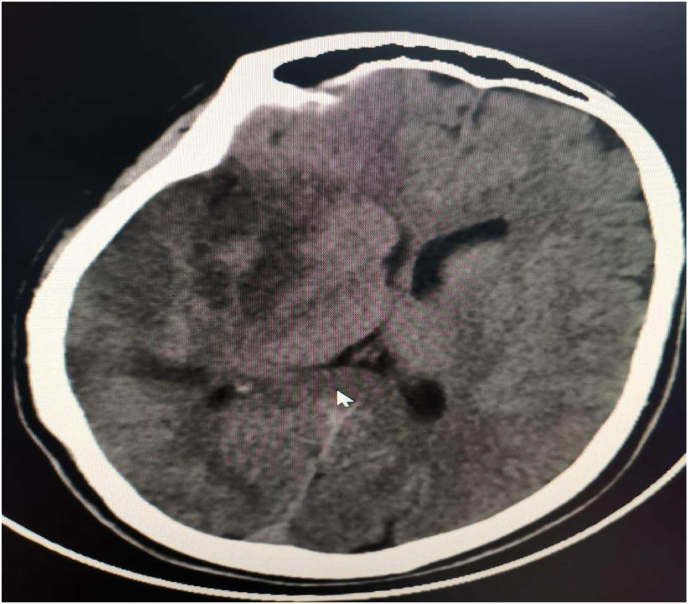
Non-contrast CT brain showed right tempro-parietal hypodense area denonting ischemic insult.
